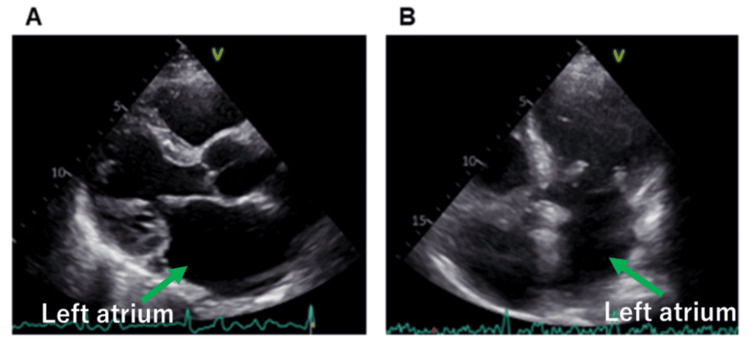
Transthoracic echocardiography revealed no abnormal structures in the left atrium.
